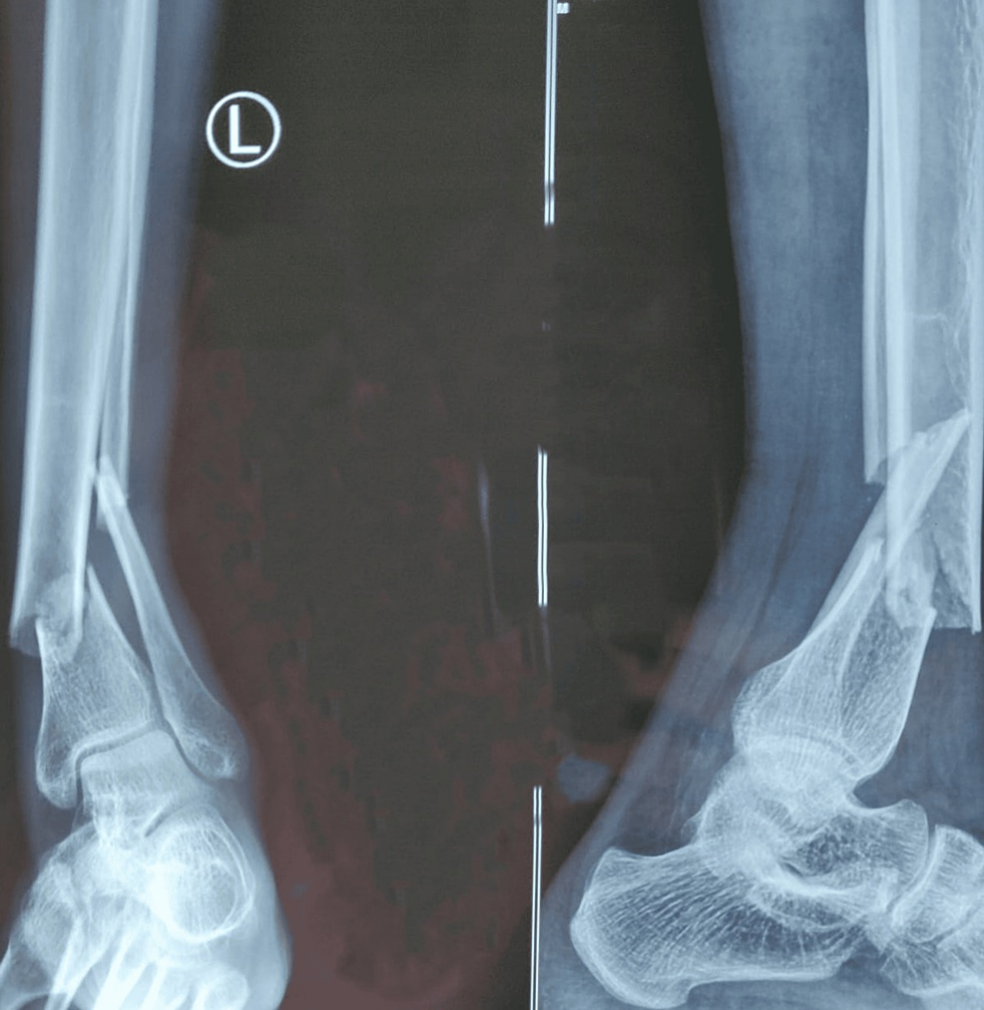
Pre-operative radiograph of distal both bone leg fracture
